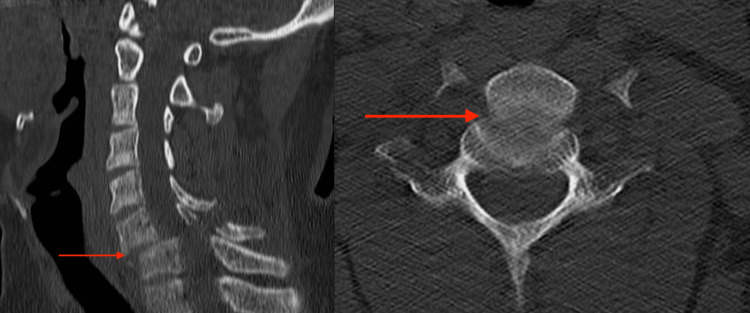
CT cervical axial (right) and sagittal (left) cuts showing fracture dislocation of C6-C7
